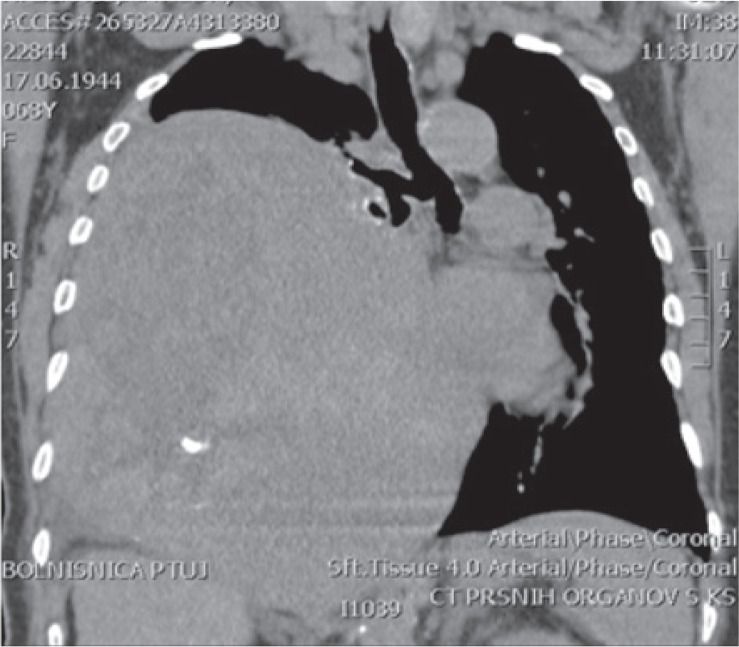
Chest CT scan - non-homogeneous, extensive tumour of the right chest side.
